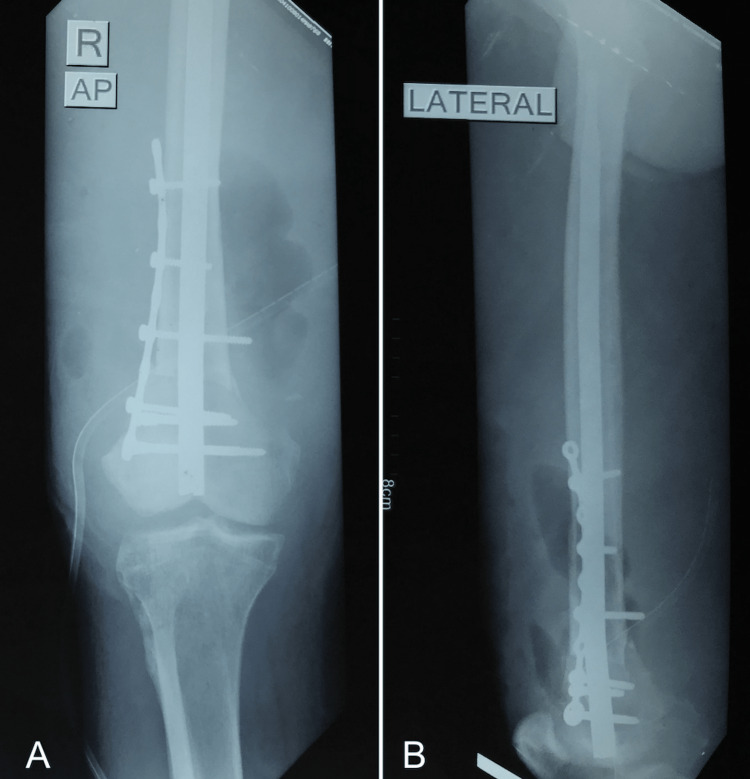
Postoperative plain radiographs of the right knee Internal fixation with a retrograde IM Nail and side plate. (A) Anteroposterior. (B) Lateral.
